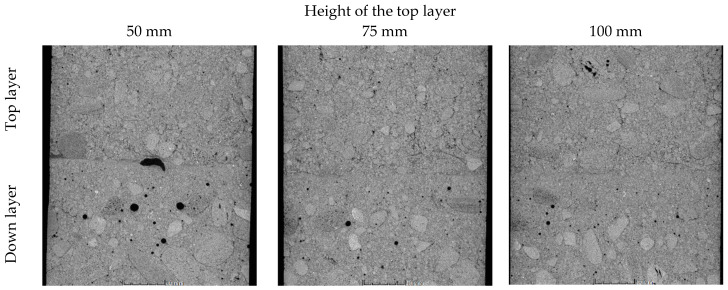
The X-ray computed tomography analysis images of the bottom and top layer interface for cores taken from the row on the opposite side from the casting point (samples C-5).
